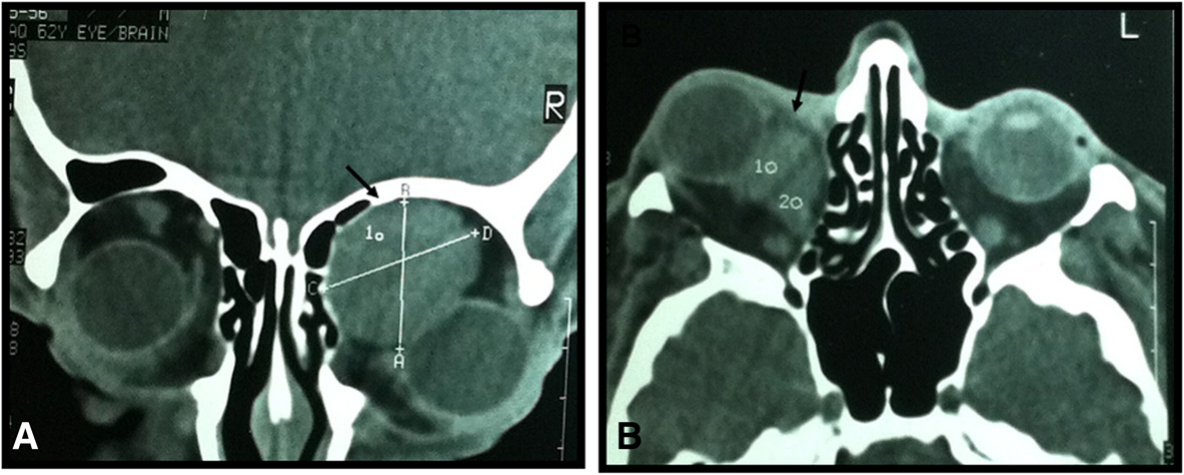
Orbital computed tomographic scans showing leiomyoma. (A) Coronal image. (B) Axial image. These computed tomographic scans reveal a well-defined soft tissue mass in the anterosuperior aspect of the right orbit (arrows in A and B). The mass is displacing the eyeball anteroinferiorly (A). The lesion reveals mild enhancement with intravenous contrast dye and is displacing the medial rectus muscle laterally (B). The left eyeball and rectobulbar spaces are normal.
